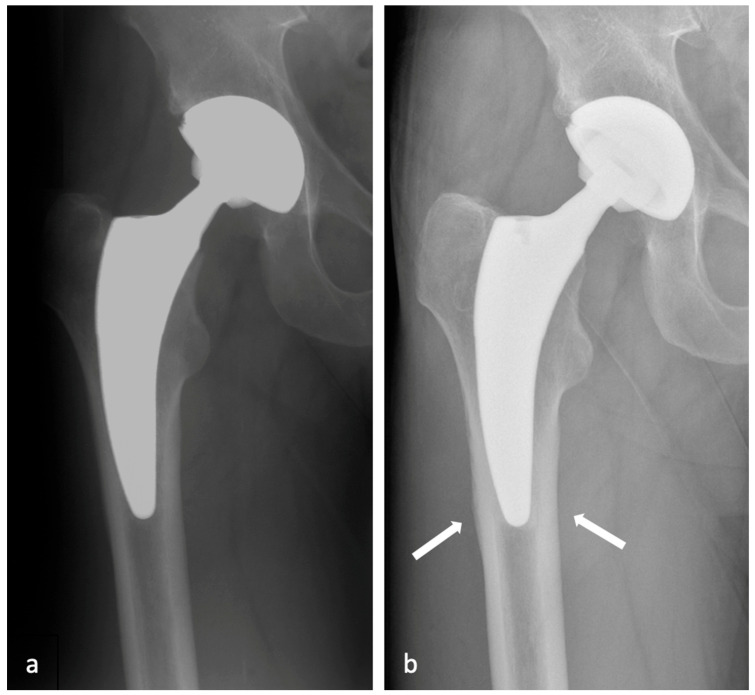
(a,b) X-rays taken 5 days postoperatively (a) in a 57-year-old male and after 11 years (b) of a representative case of cortical hypertrophy typically in zones 3 and 5 according to Gruen.
